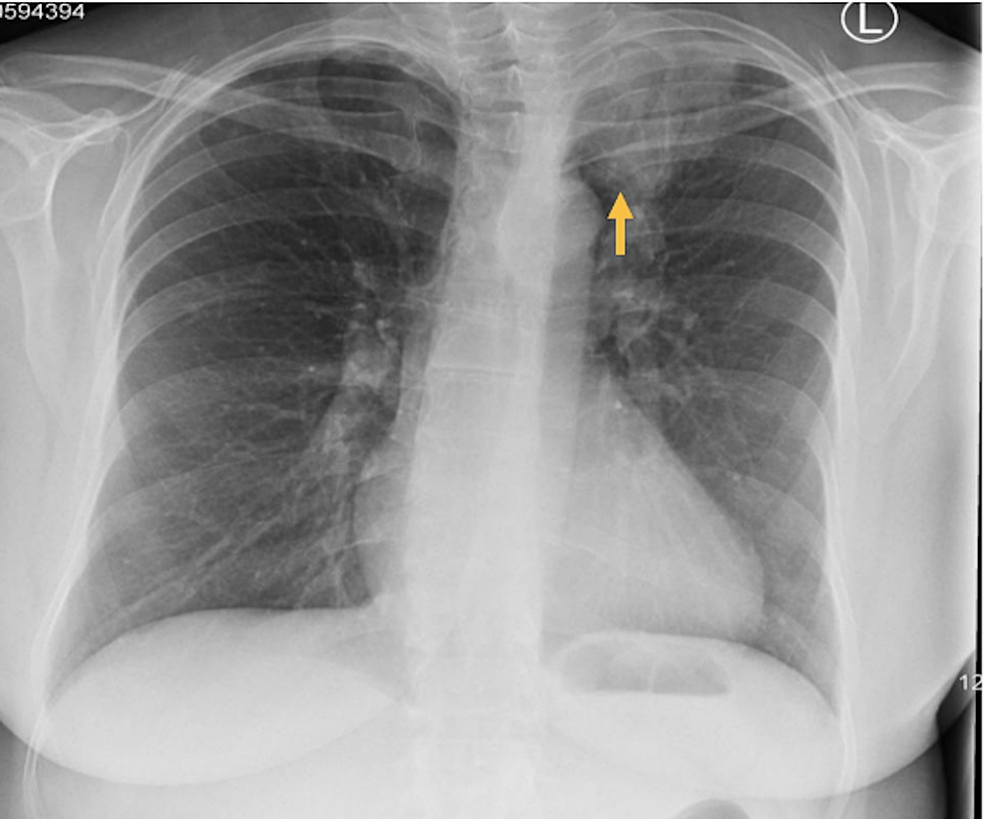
Chest x-ray showing opacification of left lung apex with slightly prominent upper left hilum (arrow)
